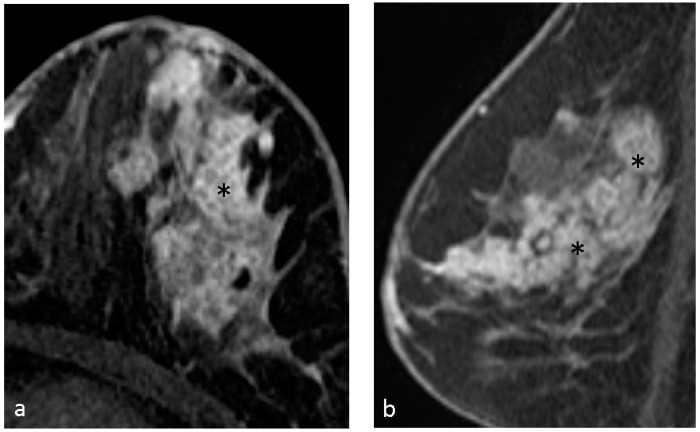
A 55-year-old woman with left breast palpable lesion for several years and with nipple serous discharge for 2–3 weeks. Axial (a) and sagittal (b) T1-weighted contrast-enhanced MR imaging shows clustered ring NME lesion (*) with segmental distribution. Pathological results of left breast revealed ductal carcinoma in situ.
